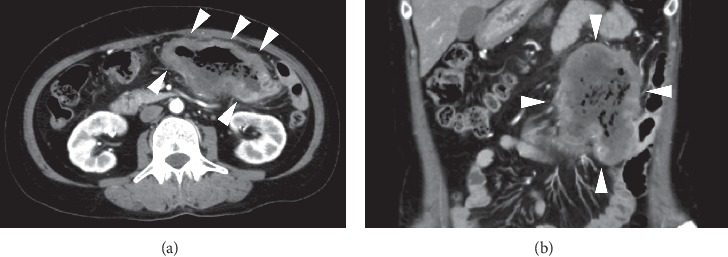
Computed tomography scanning images. Whole circumferential thickening of the jejunum is shown (arrows) (a), (b).
